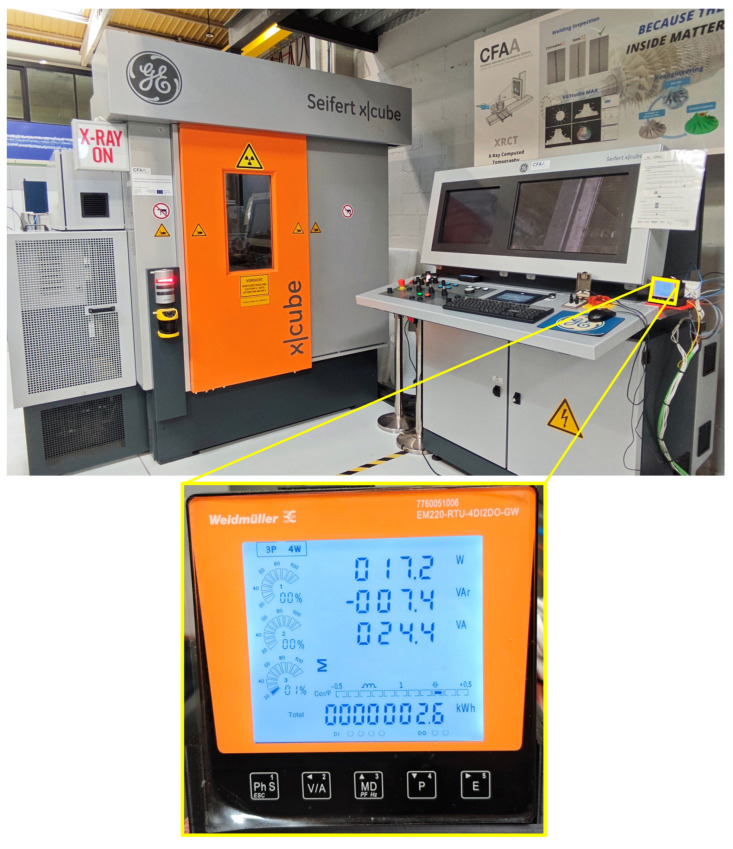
Power measurement device (Weidmüller EM220-RTU-4DI2DO-GW) integrated in the CT equipment.
